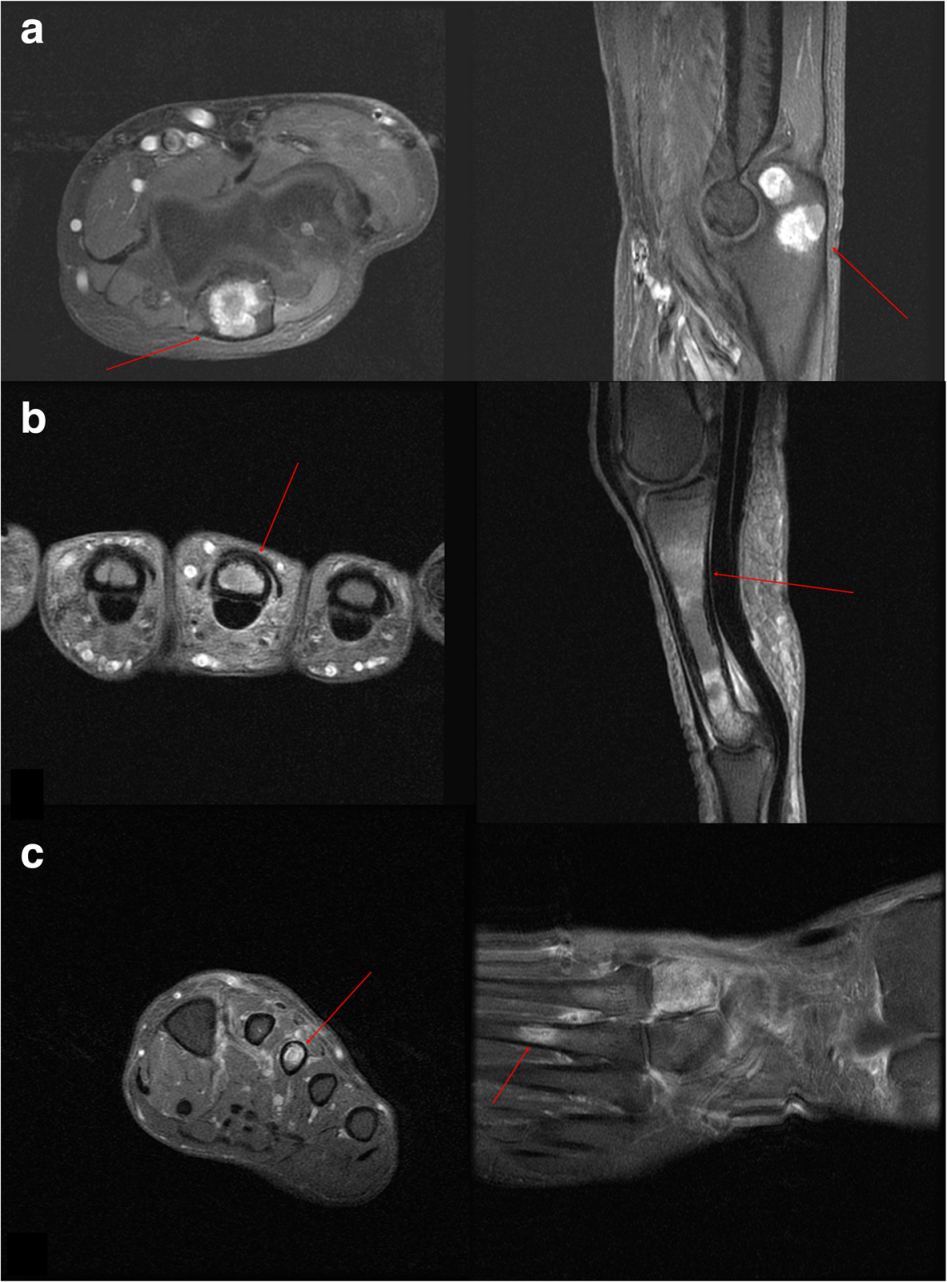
a Axial and sagittal T2-weighted magnetic resonance images show an increased signal mass in the olecranon of the left ulna (*red arrow*). b Axial and sagittal T2-weighted magnetic resonance images show an increased signal mass in the third metacarpal bone of the left hand (*red arrow*). c Axial and coronal T2-weighted magnetic resonance images show an increased signal mass in the third metatarsal bone of the left foot (*red arrow*)
